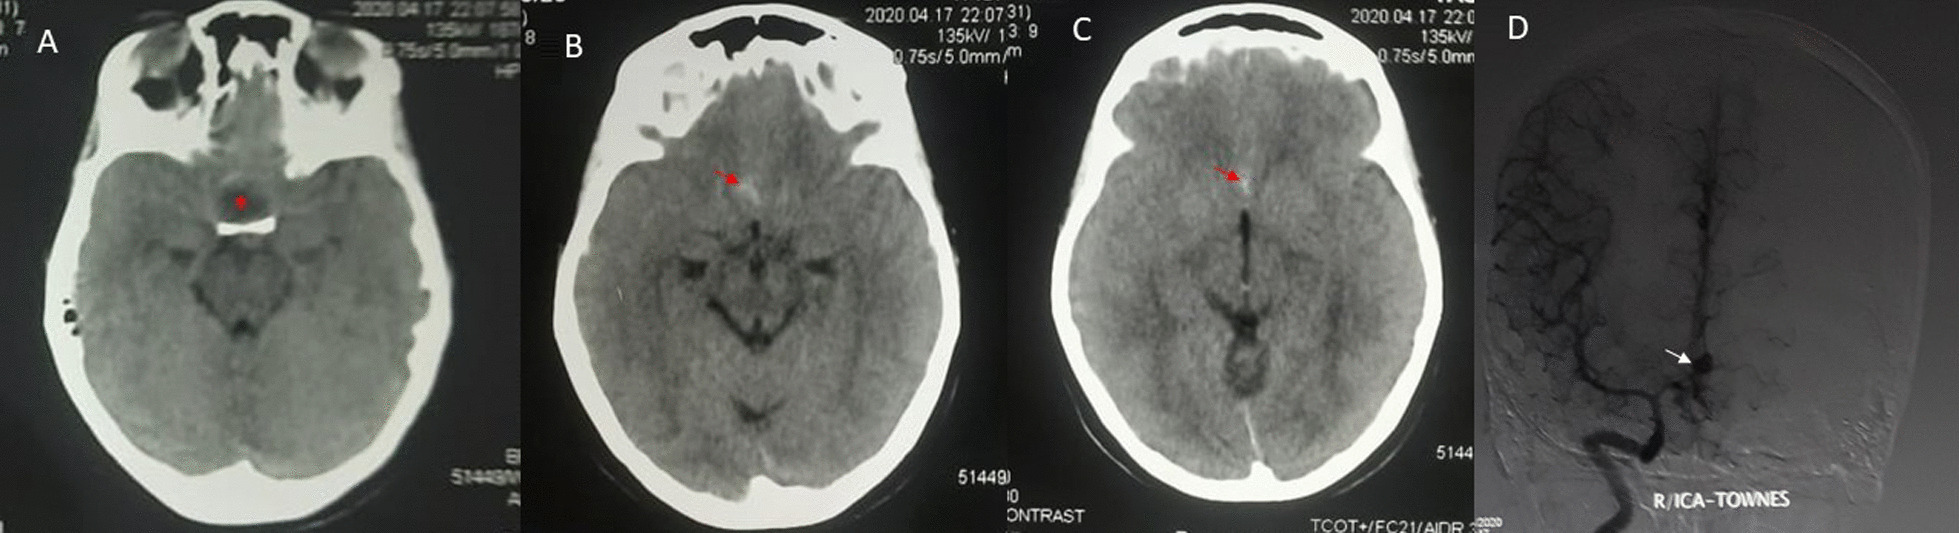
Non-contrast computed tomography of the head performed on initial presentation showing high-density signals in the basal cisterns suggestive of acute subarachnoid hemorrhage (B, C, red arrow). There is a nonspecific hypodensity in the sellar region (A, red asterisk). Cerebral digital subtraction angiogram performed during the initial presentation shows a bi-lobed secular aneurysm of the anterior communicating artery with the aneurysm sac measuring 3.7 × 4 mm anteriorly and laterally (D, white arrow)
